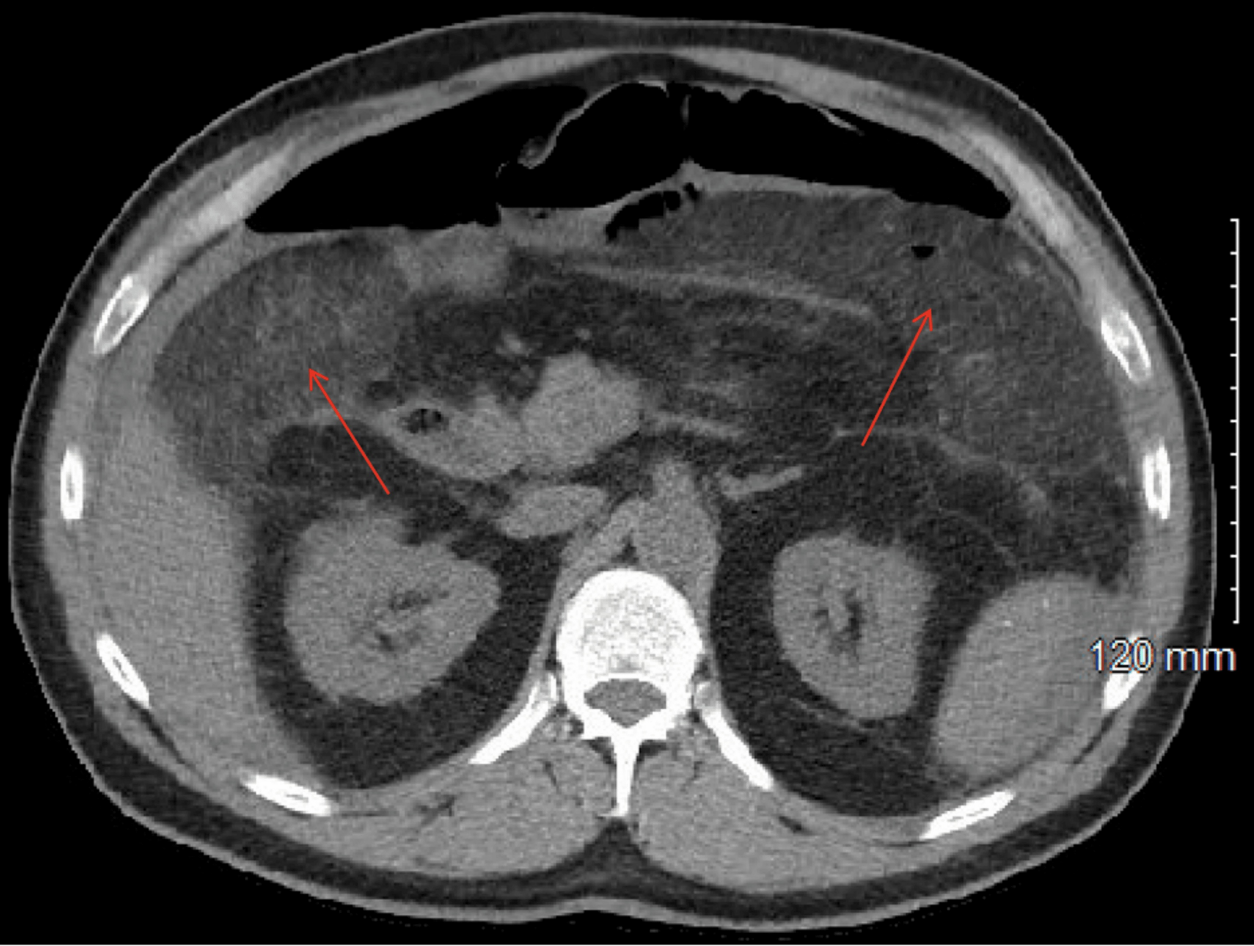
CT scan of the abdomen and pelvis without contrast (axial view), highlighting notable postoperative findings. The image reveals mesenteric stranding, which is possibly postoperative in nature (indicated by the red arrows). This mesenteric stranding may suggest inflammatory or ischemic changes related to the recent surgical intervention.
